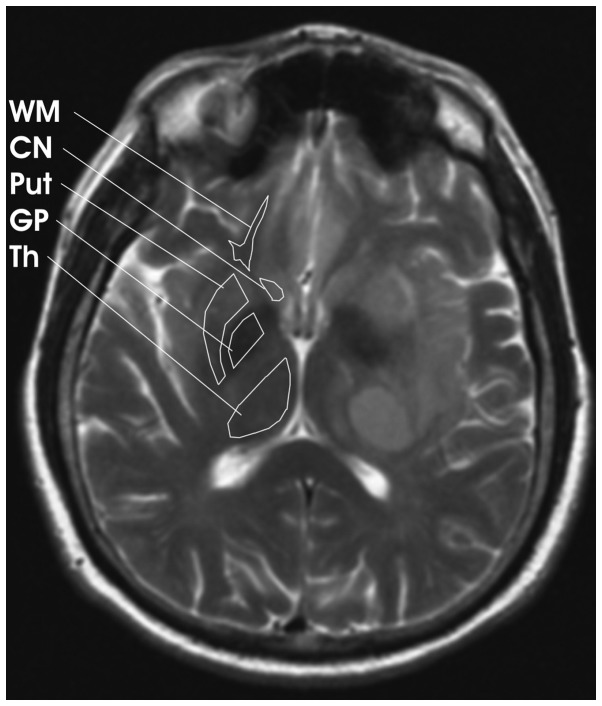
A T_2_-weighted magnetic resonance image of a patient with a high-grade glioma IV (glioblastoma multiforme in the left hemisphere). The regions of interest in the white matter (WM), caudate nucleus (CN), putamen (Put), globus pallidus (GP) and thalamus (Th) contralateral to the lesion are highlighted.
